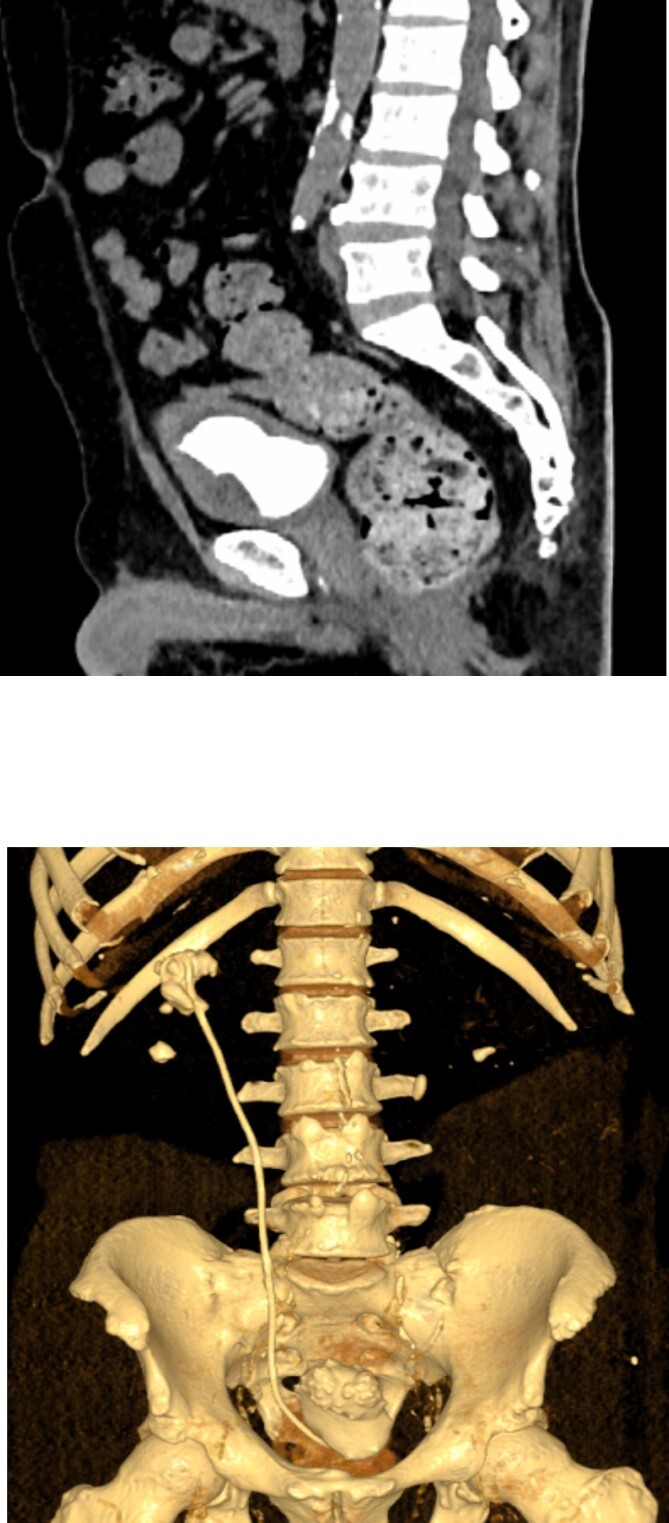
Non-contrast CT scan showed giant bladder stone profoundly embedded in the distal pigtail of the DJ stent.
